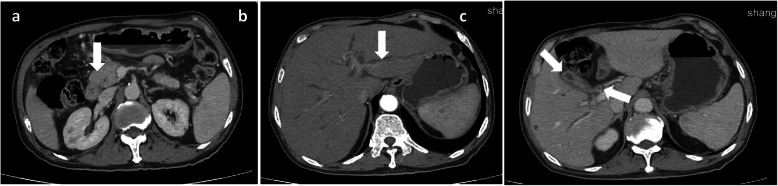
Abdominal enhancement CT suggested lesions in the intestinal tract. a There was a mass at the pancreatic head, indicated by arrow. b Intrahepatic bile duct was dilated, indicated by arrow. c The gallbladder wall (left arrow) and the wall of the upper end of the common bile duct (right arrow) were thicken
